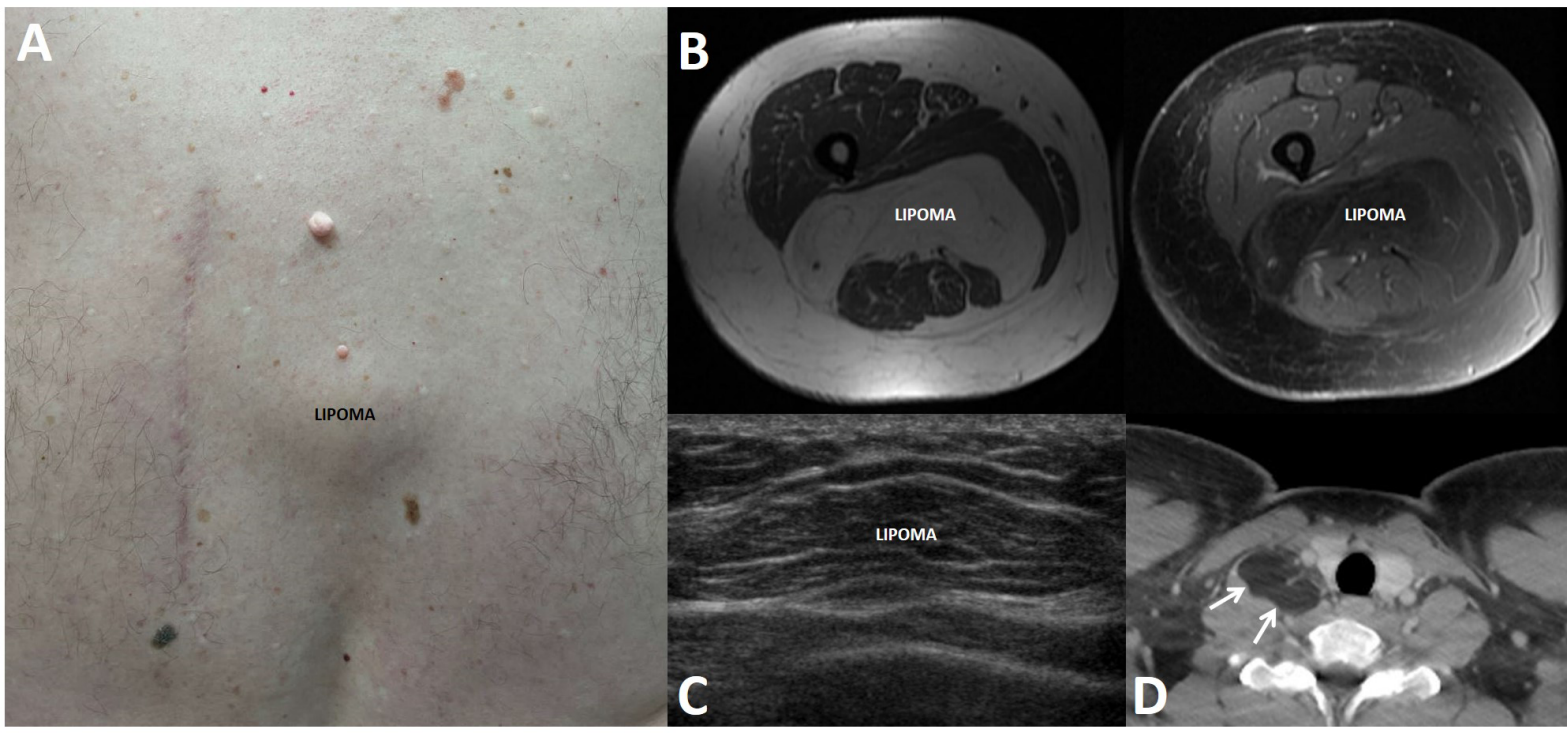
Lipomas in patients with multiple endocrine neoplasia type 1. Lipomas are benign tumors made of mature adipocytes that can arise from anywhere that fat is located. (A) 62-year-old man with a subcutaneous lipoma located over the spine in the mid back. Also seen is an adjacent surgical scar related to the prior resection of a large lipoma and scattered collagenomas. (B) 49-year-old woman with multifocal intramuscular lipomas, including a large posterior thigh lipoma as seen on axial T1-weighted pre- (left panel) and post-contrast (right) magnetic resonance imaging. (C) 16-year-old boy with a mobile soft tissue mass arising over the lower anterior chest. Ultrasound showed a 3.7 cm lesion consistent with lipoma. (D) 25-year-old man with a 3.6 cm lipoma (arrows) in the right scalene muscle, adjacent to the right thyroid lobe, as seen on contrast-enhanced computed tomography.
